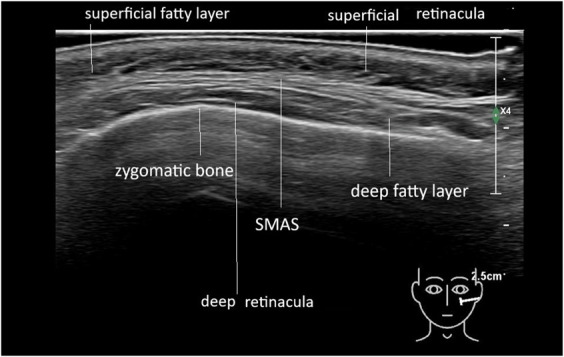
Ultrasound images of normal skin, superficial fat, SMAS and deep fat, the retinacula cutis superficialis and profundus. SMAS, superficial musculo-aponeurotic system.
